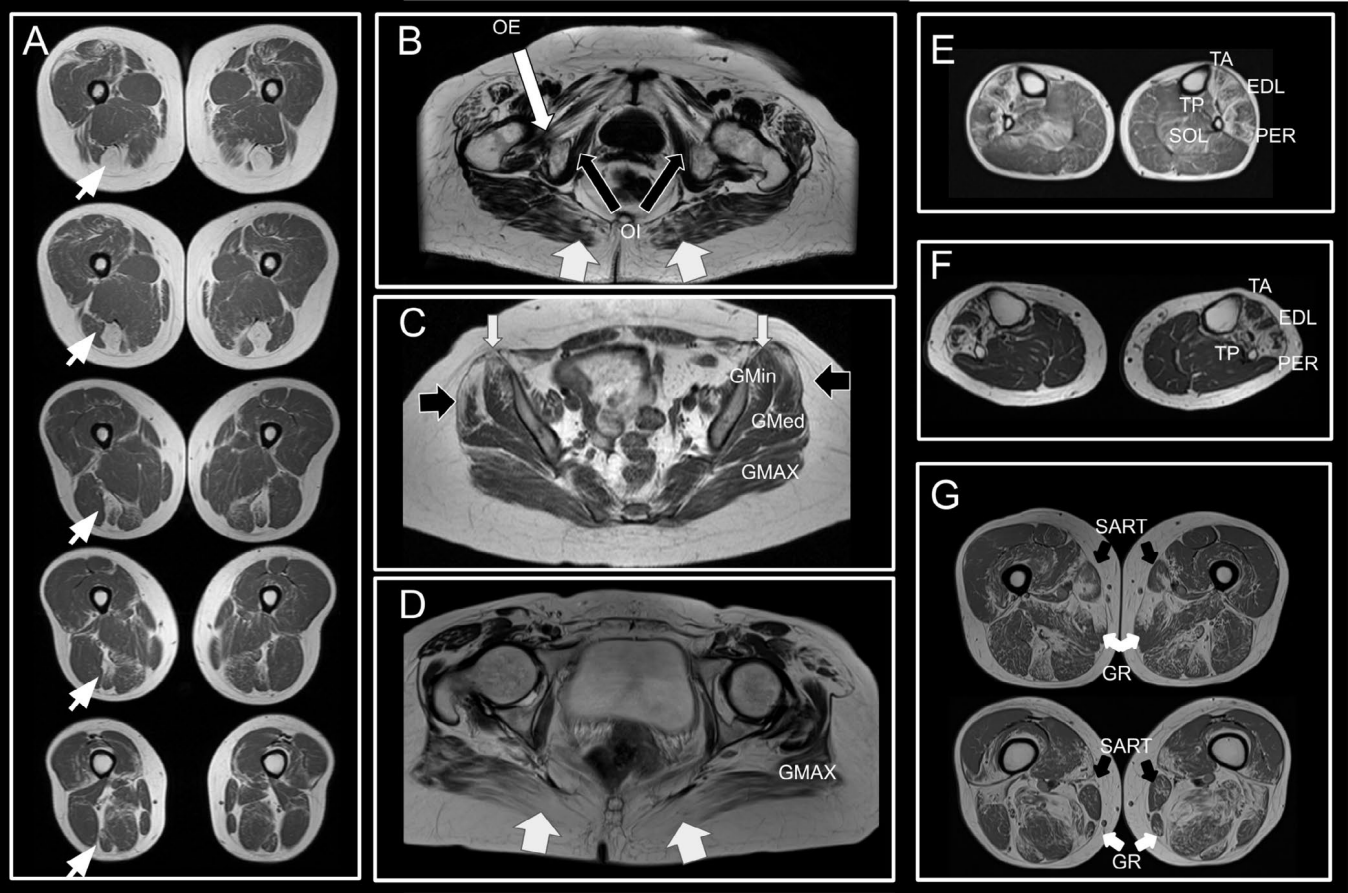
Selected MR images from different patients with HMERF. (A) Axial thigh images from proximal to distal are shown to demonstrate the typical involvement of semitendinosus muscle (white arrow in the left leg) in the disease. Complete fat replacement occurs in the proximal part of the muscle, whereas distally and posteriorly, muscle bulk is preserved. (B) Lower axial pelvic MR image (IDEAL) that shows the preservation of the obturator internus muscle in comparison to the obturator externus. Notice also the involvement of the medial part of gluteus maximus. (C) High axial pelvic view to glutei muscles. Notice the medial and anterior involvement of gluteus minimus (white arrows) and the anterior involvement of gluteus medius (black arrows). (D) Typical gluteus maximal involvement with severe medial involvement and preservation of the middle part. (E, F) Severe involvement of anterior compartment in two patients. Notice the heterogeneous intramuscular involvement of muscles. (G) Thigh images of the same patient. Notice the distal preservation of gracilis and sartorius in comparison to proximal involvement.
